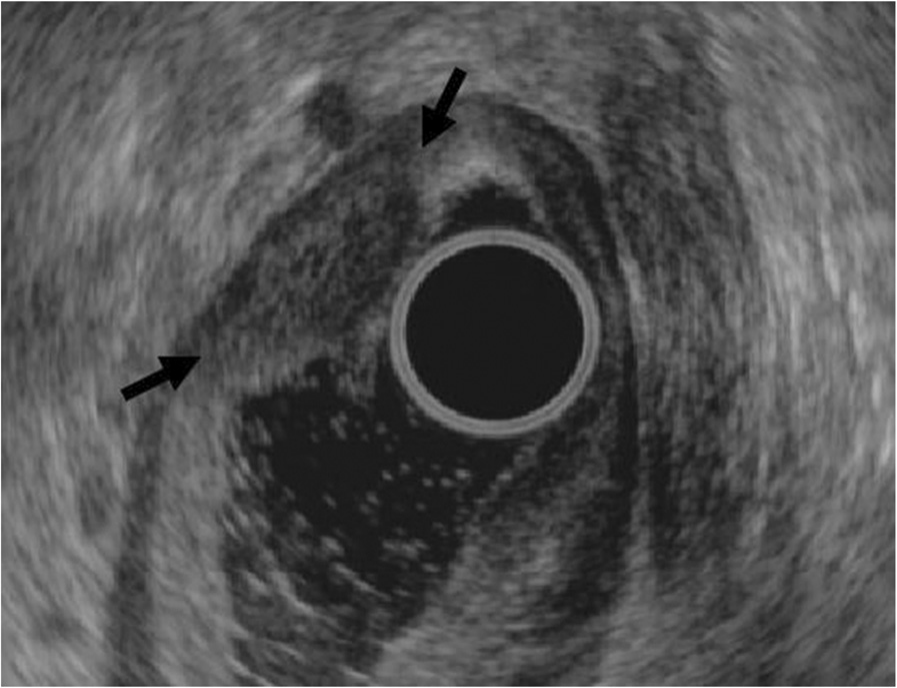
Endoscopic ultrasonography showing a hypoechoic mass without clear margins (arrows) in the submucosa.
